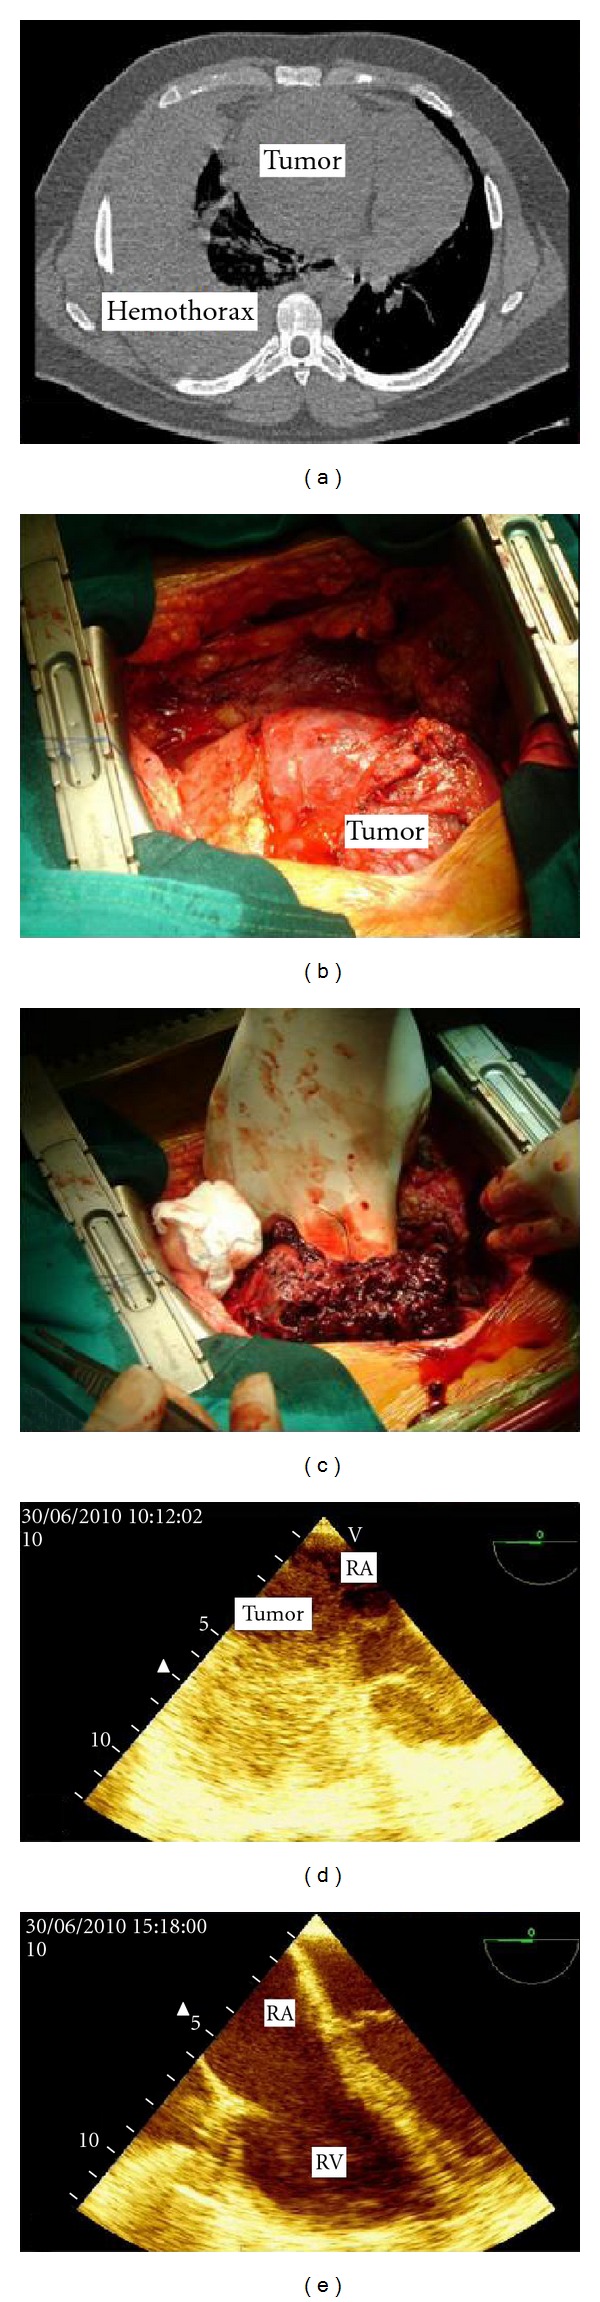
(a) Chest CT; (b) Tumor in RA displayed sternotomy (c) extensive necrosis and hemorrhage of the tumor to manipulation; (d) peroperative transesophageal echocardiogram, showing compression of RA, resolved after resection of the tumor (e) RA: right atrium; RV: right ventricle.
